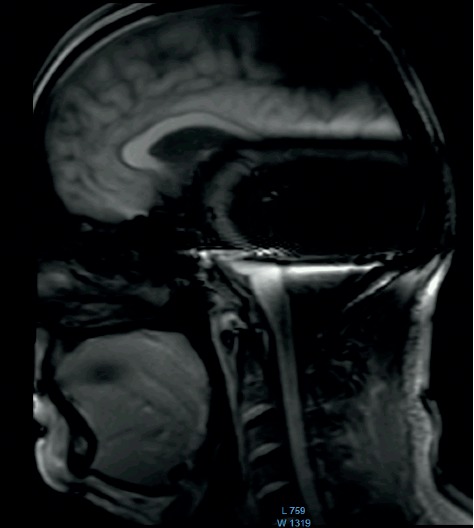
MRI survey pattern of the sagittal plain of a 3 T scanning of an Advanced Bionics 3D Implant.
